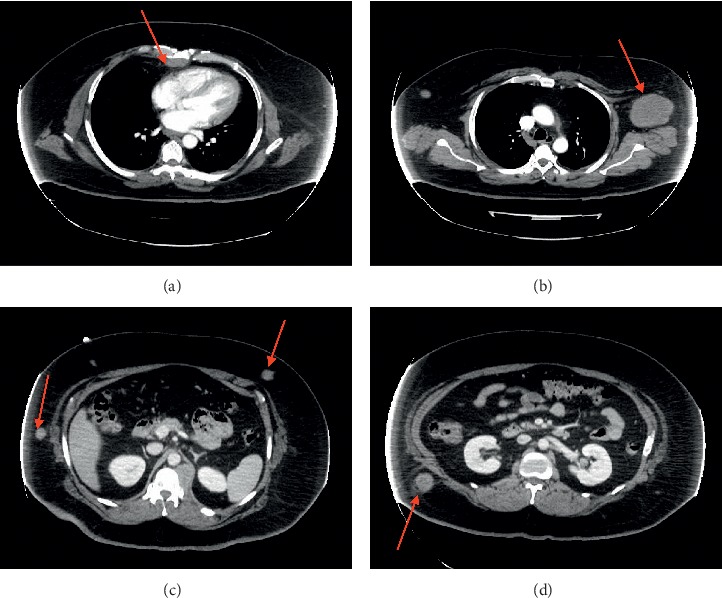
CT scan of the chest and abdomen showing multiple soft-tissue masses involving the anterior mediastinum (a), left axillary, (b) and abdominal subcutaneous tissue (c and d).
